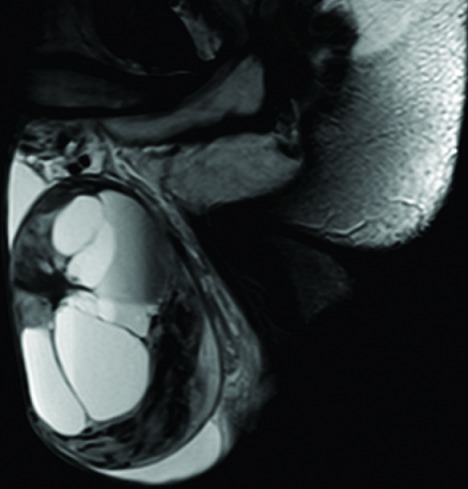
Sagittal T2- weighted MRI of the scrotum shows large intratesticular multilocular complex cystic lesion. Some of the cysts showed fluid fluid levels. The remaining testicular tissue appears of heterogeneous hyperintense signal with mild surrounding hydrocele
